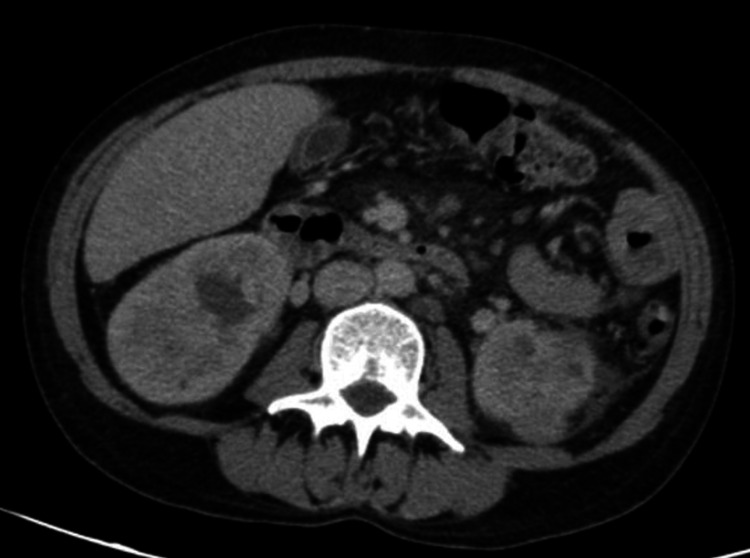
Abdominopelvic CT scan showing no renal abscess recurrence CT: computed tomography
